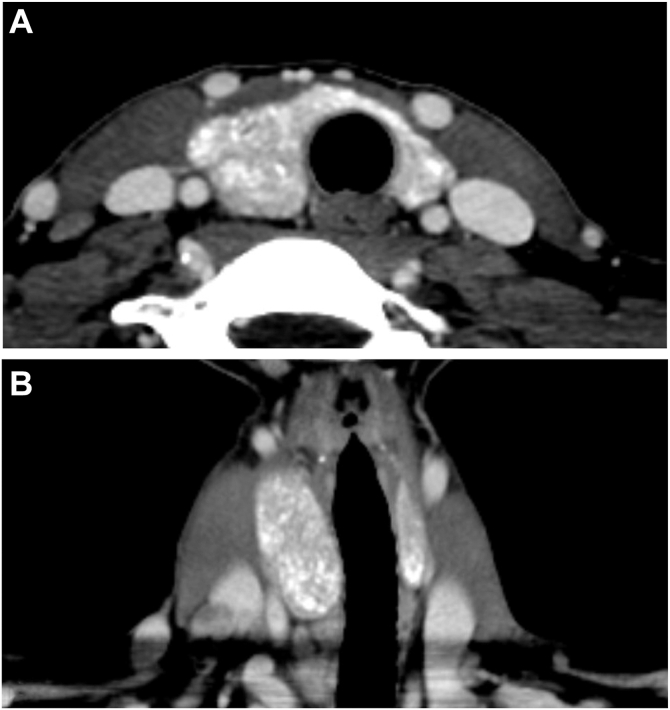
Neck computed tomography (CT) image in the (*A*) axial view and (*B*) coronal view showing asymmetric, heterogeneous mass-like enlargement of the *right* thyroid lobe.
